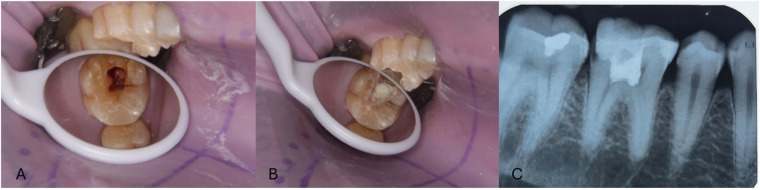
Clinical and postop IOPA radiographic representation of pulpotomy with the use of biodentine. (A) Full pulpotomy and, (B) Application of Biodentine at pulpotomy site, (C) Post IOPA Radiograph following Pulpotomy procedure.
